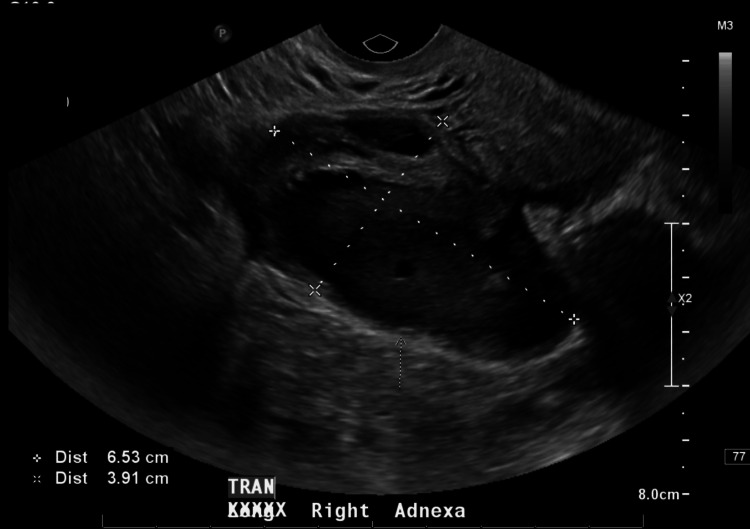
Transvaginal ultrasound nine weeks after the discharge, revealing a stable tubo-ovarian complex measuring 6.5 x 3.9 cm. No ascites were present.
